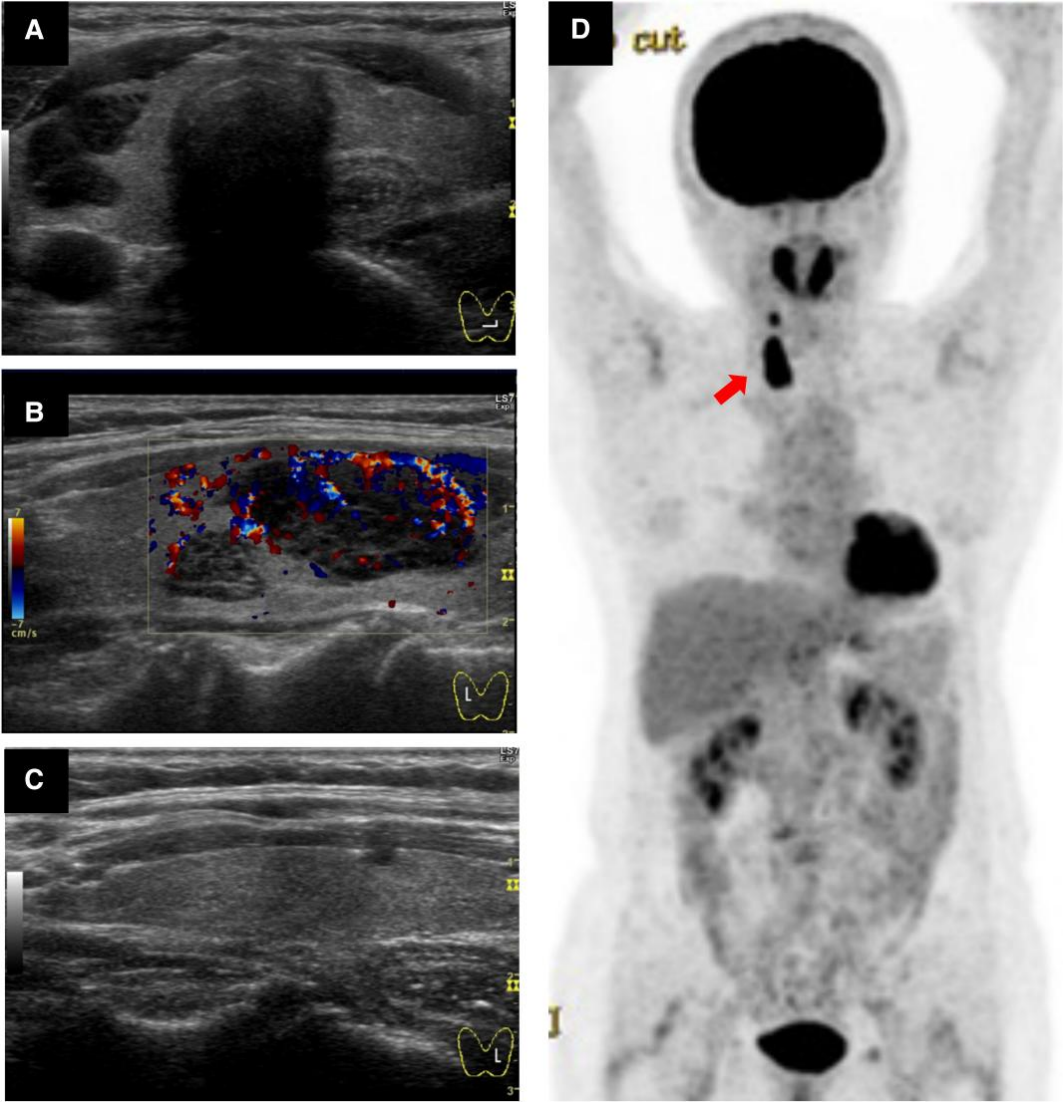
Imaging at the first surgery. (A-C) Ultrasound examination showed hypoechoic masses in the right thyroid lobe, measuring 25 mm × 19 mm and 11 mm × 9 mm. The border was well-defined, with the margins exhibiting a broccoli-like growth pattern. The left lobe appeared normal. (D) Fluorodeoxyglucose positron emission tomography revealed intense uptake corresponding to the masses in the right thyroid lobe (SUVmax 14.0), with no abnormal uptake elsewhere.
